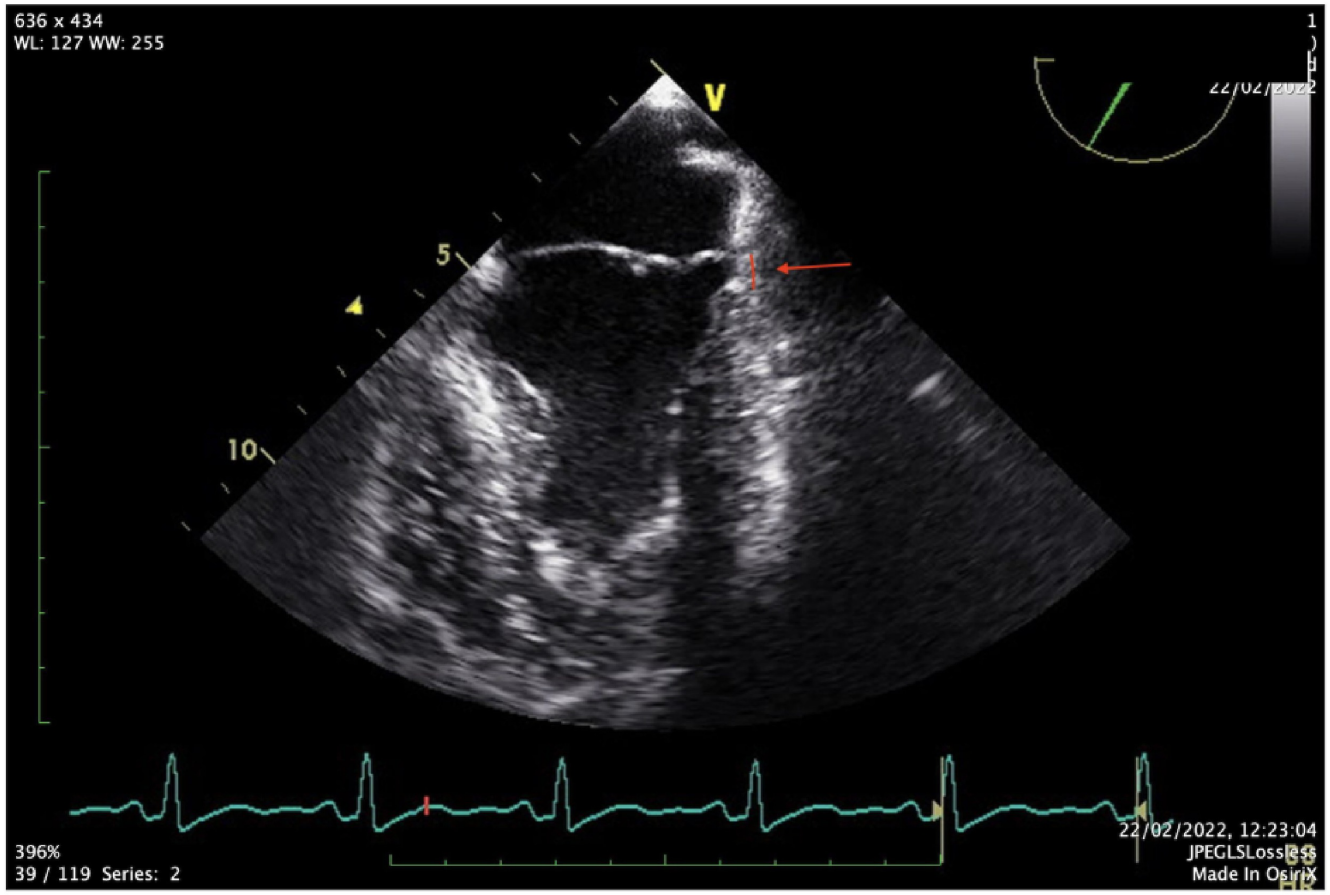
Transesophageal view of MAD (red line and red arrow).
